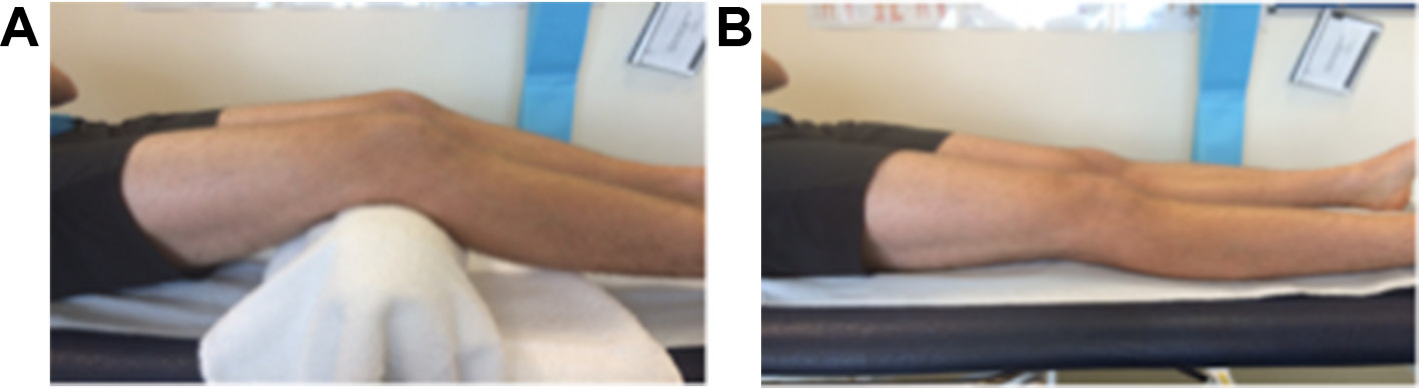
(A)Standardised position for US imaging of the patella tendon (position 1). (B) Standardised position for US imaging of the patella tendon (position 2). US, ultrasound.
